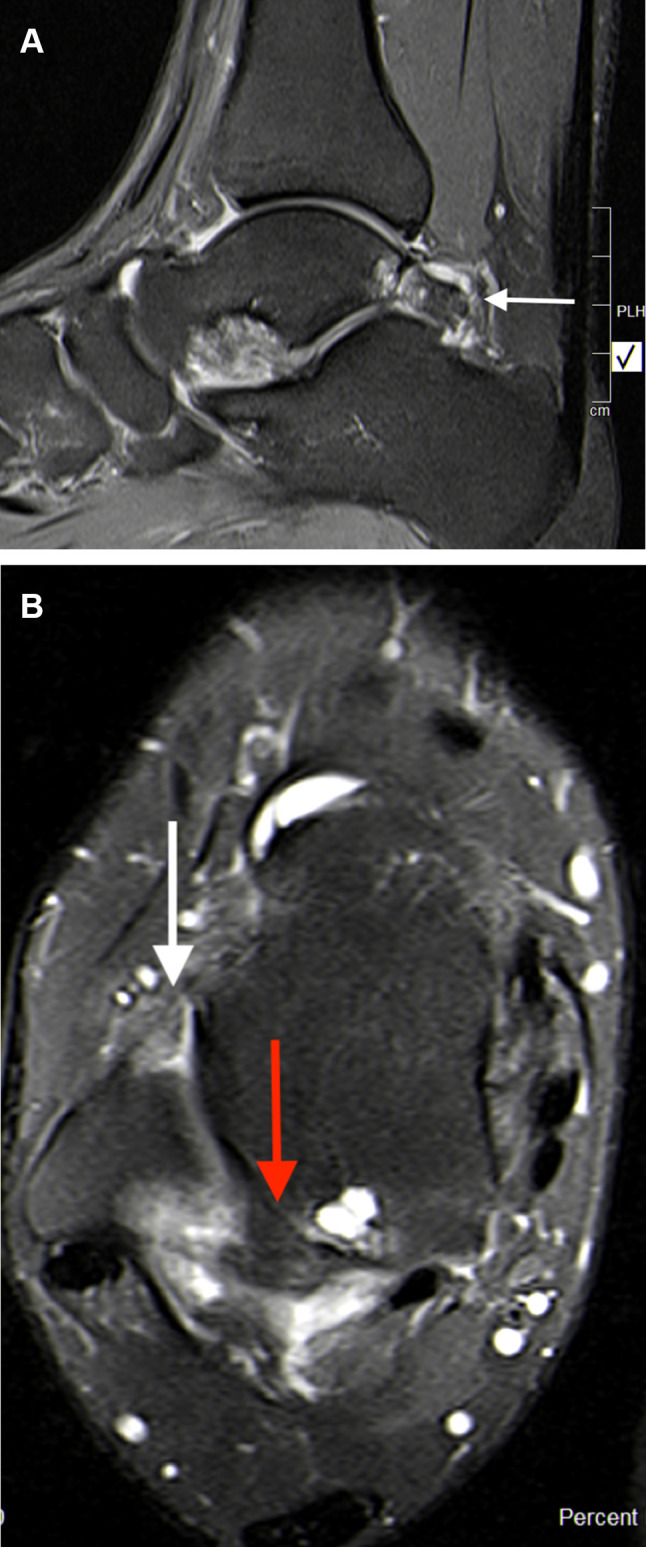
a Sagittal T2 MRI image depicting inflammatory signs and bony oedema over the os trigonum complex (white arrow). b Axial T2 MRI image depicting inflammatory signs and bony oedema over the os trigonum (red arrow) in an athlete with a combined chronic injury to the lateral ligament complex (white arrow)
